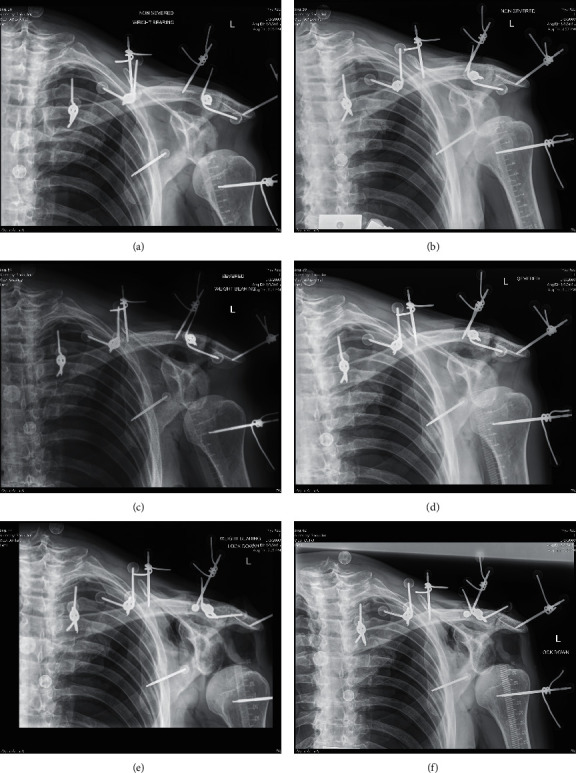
Radiographs were used to measure the coracoclavicular distances in the following states: “native” stressed (a), “native” unstressed (b), “severed” stressed (c), “severed” unstressed (d), “reconstructed” stressed (e), and “reconstructed” unstressed (f).
